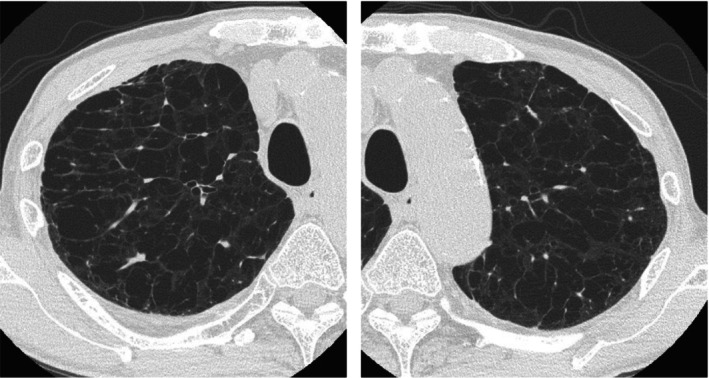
The axial view of the chest computed tomography (CT) scan acquired with a 1‐mm slice thickness at 120 kVp and 350 ms revealed severe emphysema.
